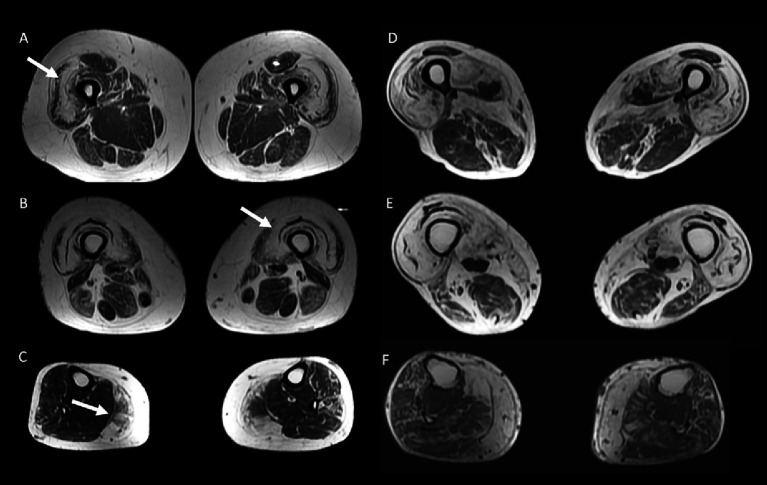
50 year-old female with inflammatory myopathy with mitochondrial pathology (IM-Mito) (A–C) presents axial fast spin-echo T1-weighted Whole-Body Muscle Magnetic Resonance Imaging (WBMRI) showing moderate-severe fatty infiltration in quadriceps muscle with relatively spared rectus femoris muscle compared to other quadriceps muscles (A,B) and severe fatty infiltration in medial gastrocnemius muscle (C). Axial fast spin-echo T1-weighted WBMRI in a 78 year-old male with inclusion body myositis (D–F) shows similar imaging appearance compared to IM-Mito shown in (A–C).
